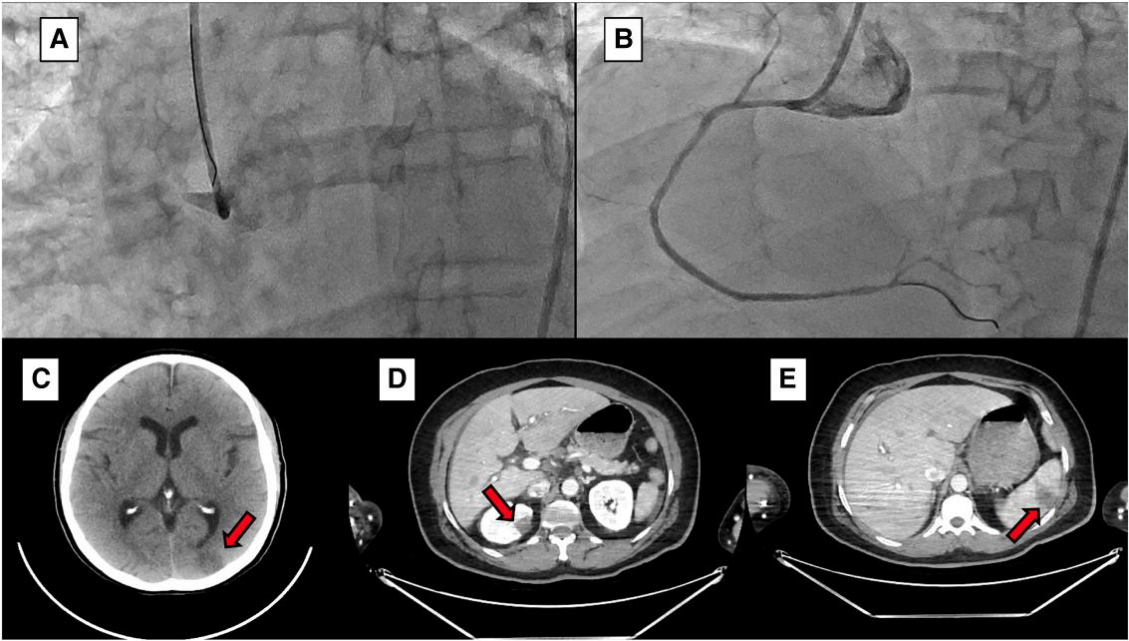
Angiographic evidence of an occluded right coronary artery at the ostium (*A*) and reperfusion after guidewire crossing of thrombus (*B*); computed tomography angiography evidence of embolism in the brain (*C*), kidney (*D*), and spleen (*E*).
